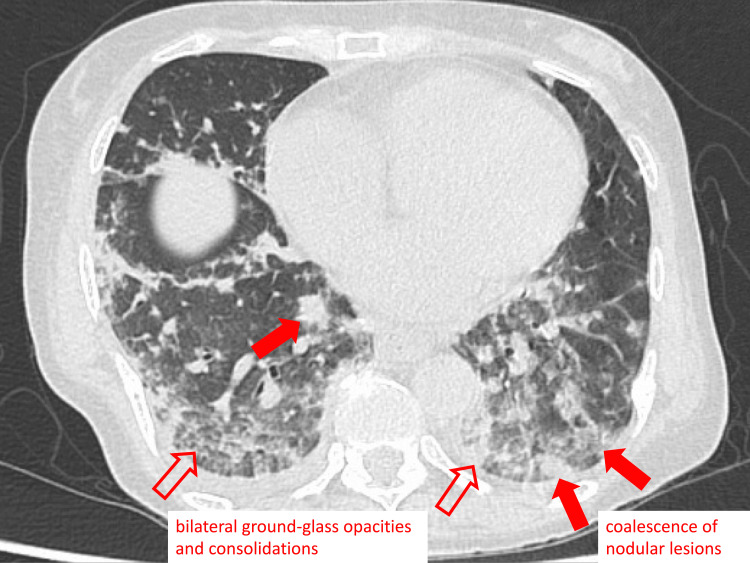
Chest computed tomography imaging on the third day of admission Chest computed tomography revealed diffuse bilateral ground-glass opacities, coalescence of nodular lesions, and consolidation.
